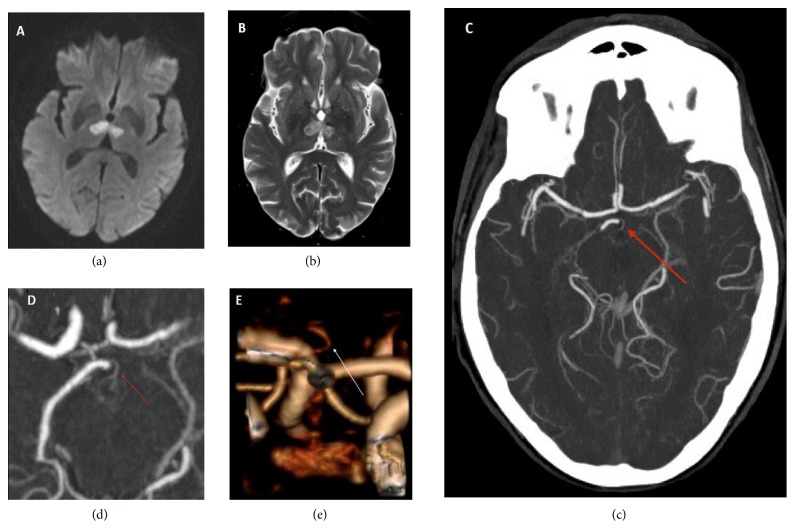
MRI brain diffusion weighted imaging series (a) and T2 (b) demonstrating bilateral paramedian thalamic infarcts. CTA vessel study. (c) Axial-Maximum Intensity Projection (MIP); (d) axial-3D-MIP; and (e) reconstructed 3D image demonstrates an Artery of Percheron (arrow) arising from the right Posterior Cerebral Artery P1 segment.
